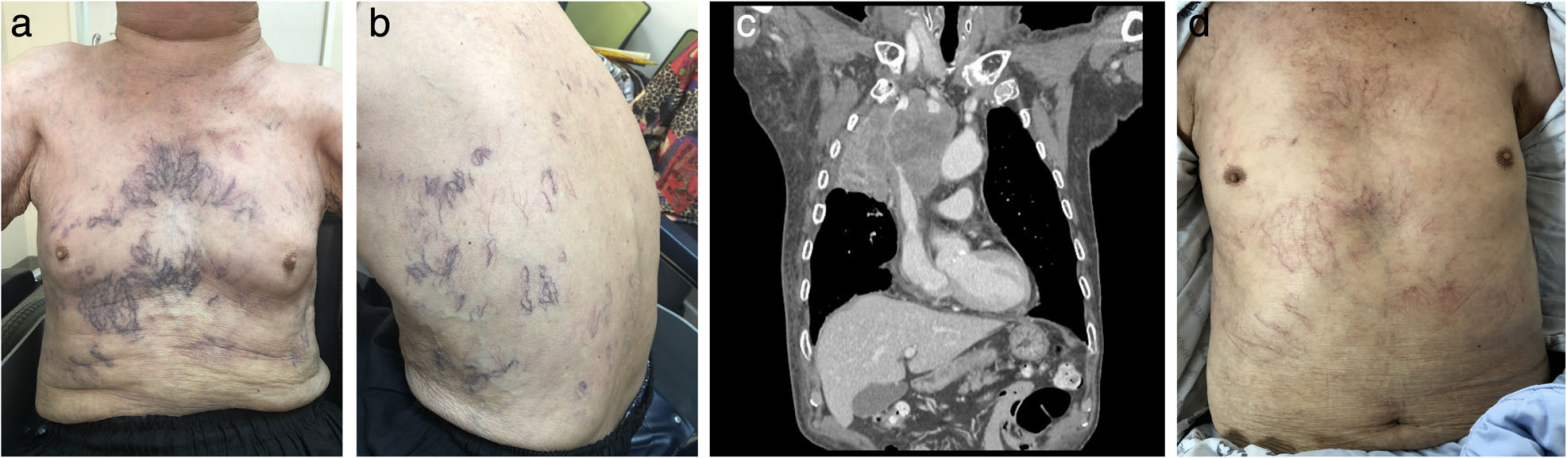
Anterior and Lateral Chest (a, b) and Computed Tomogram (c) before chemotherapy, and Chest (d) after chemotherapy.
